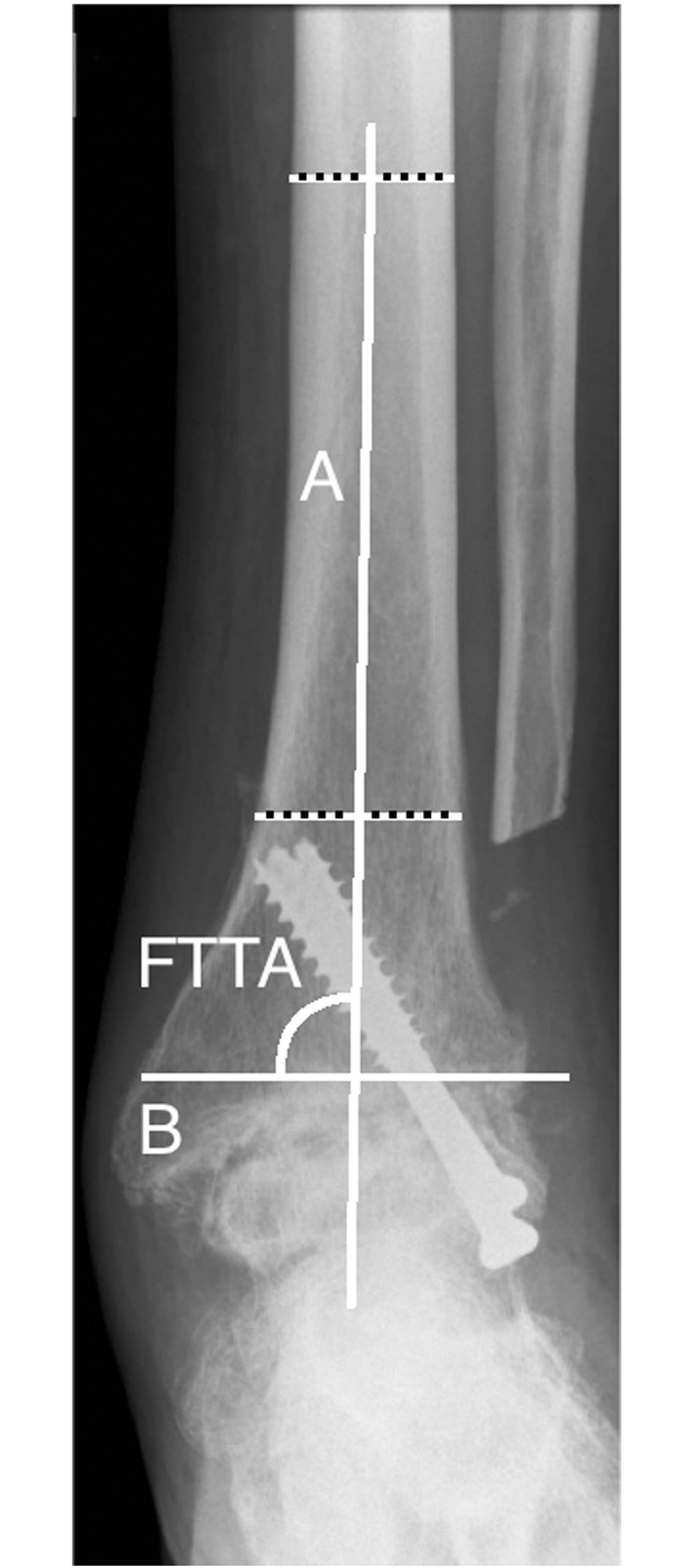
Anterior-posterior radiograph ankle arthrodesis. Anterior to posterior ankle radiograph with the measurement of the frontal tibiotalar angle (FTTA). The FTTA is the superomedial angle between the two axes A and B. A = longitudinal axis of the tibia, created by connecting two points in the middle of the proximal and the distal tibial shaft (according to method B). B = axis of the talus, defined by a line drawn through the talar shoulders.
